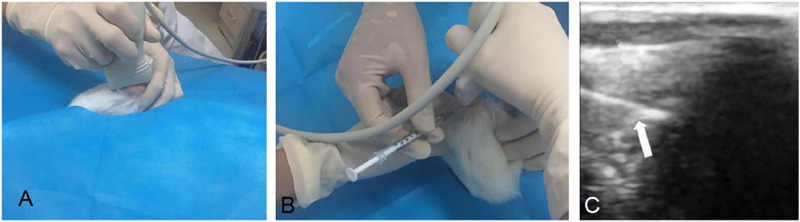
Ultrasound guided intra-articular injection. Knee joint of rabbits were scanned longitudinally with ultrasound (A); transverse scan of knee joint parallel to the needle feeding direction (B); ultrasound of the needle (white arrow) punctured into the articular cavity (C).
